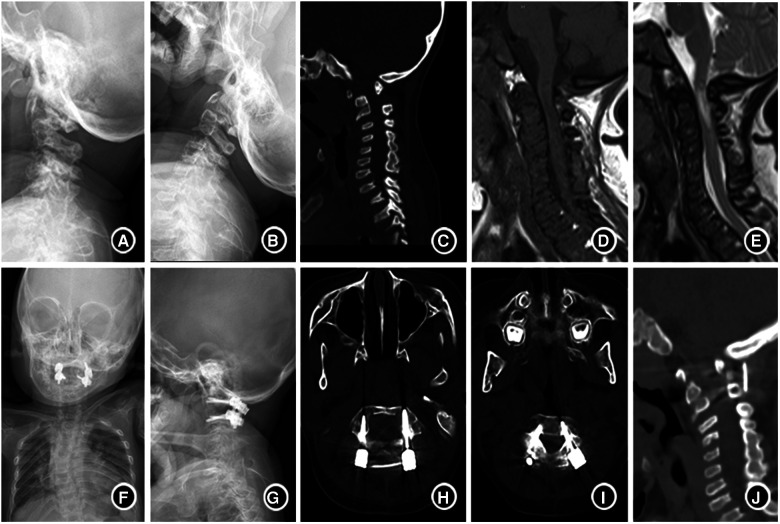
A 4-year-old boy with type IVA mucopolysaccharide disease (A,B). Lateral plain-film flexion-extension studies before the surgery showed uneven cervical bone density, flattened vertebra, atlantoaxial instability. (C) CT suggested atlantoaxial subluxation and odontoid process absence; (D,E) MRI indicated spinal canal stenosis, high cervical spinal cord compression and edema; (F,G) showed that the anatomical position of atlantoaxial returned to normal half a year after operation. (H–J). CT showed that the screw position was good, and the bone graft was fused with the atlantoaxial vertebra 6 months after operation.
